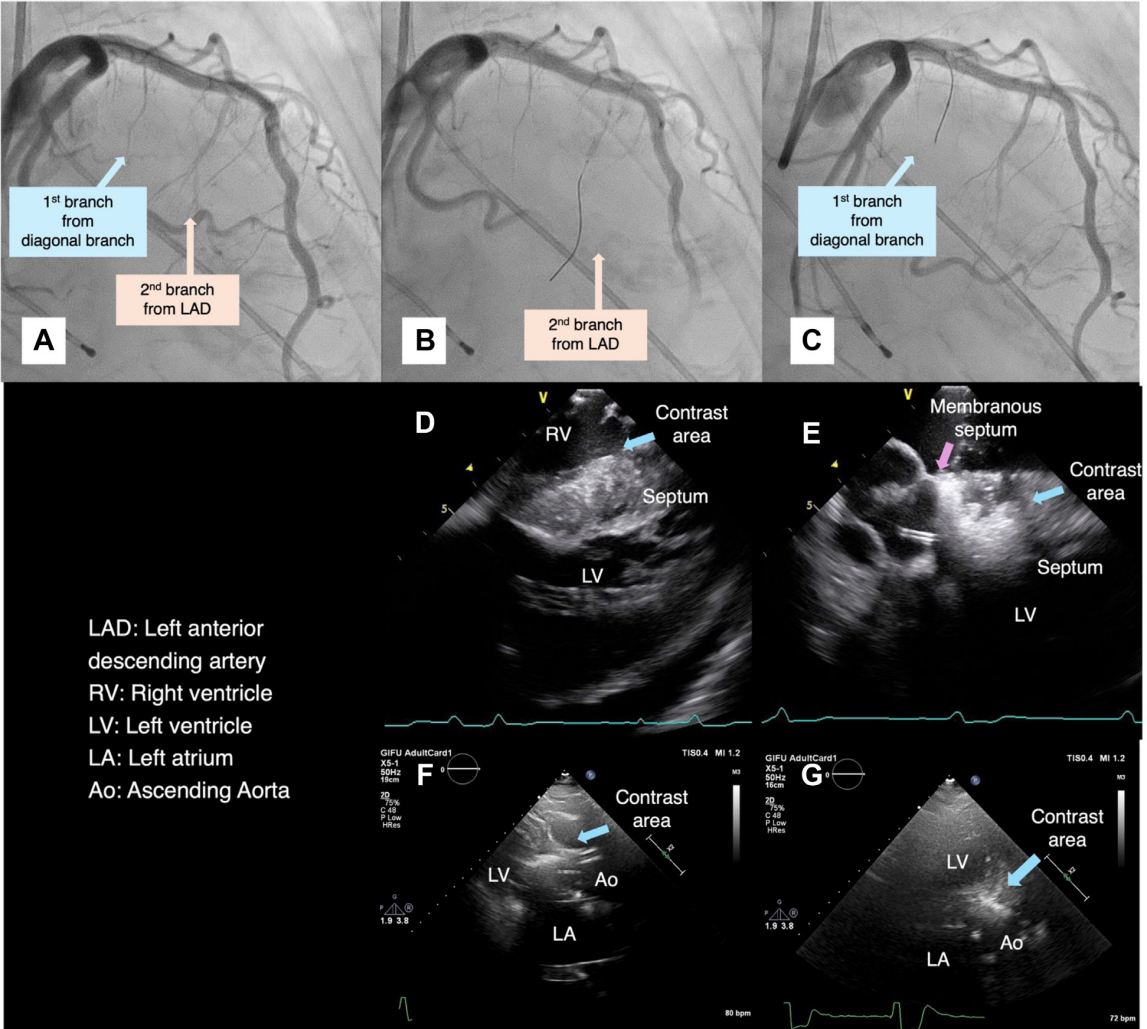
Imaging in Case 3 (A to C) Coronary angiography (A) before ablation, (B) during ballooning to the second branch from the left anterior descending artery (LAD), and (C) during wiring to the first branch from the diagonal branch. (D and E) Jugular vein intracardiac echocardiography showing (D) contrast agent injection to the second branch from the left anterior descending artery and (E) ethanol injection to the first branch from the diagonal branch. High signal intensity is observed up to the membranous septal region. (F and G) Transthoracic echocardiography. (F) Parasternal long-axis view. (G) A 3-chamber view. Compared with (D and E) the intracardiac echocardiography imaging, (F and G) the transthoracic echocardiography imaging was poor. Ao = aorta; LA, left atrium; LV = left ventricle.
